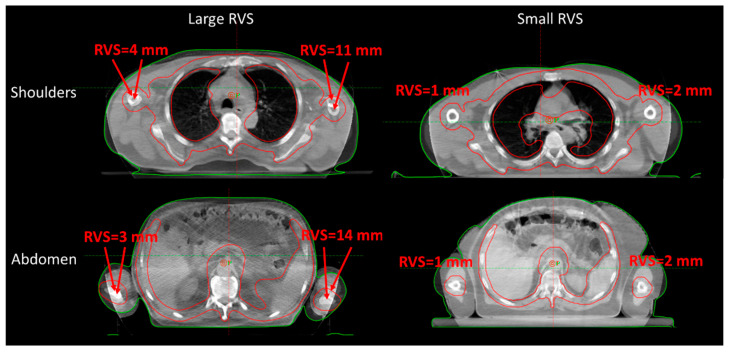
Representative CBCT-CT matching was performed for the shoulders (top) and abdomen (bottom) isocenters. The RVS was measured as the maximum center distance between the bone marrow of the extremities. Large (left) and small (right) RVS values are reported. RVS = residual vector shift.
